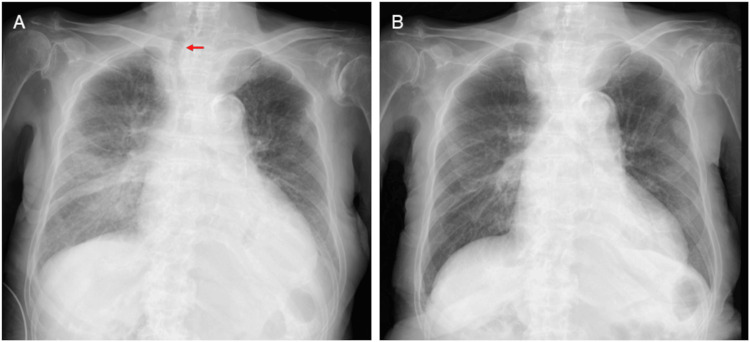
Serial chest radiographs. Initial chest radiography shows cardiomegaly and pulmonary congestion, together with rightward deviation of the trachea, presumably due to a thyroid tumor (A). Follow-up chest radiography on hospital day 20 demonstrates marked improvement in pulmonary congestion (B).
